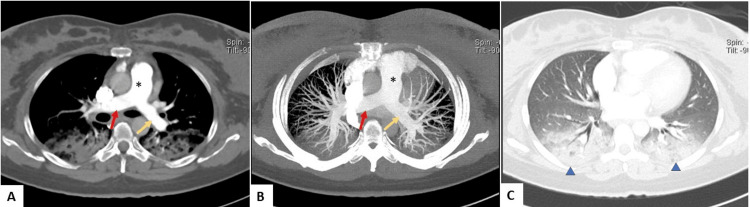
CT imaging: pulmonary angiography. (A) Axial pulmonary angiogram. (B) Axial maximum intensity projection. (C) Axial lung window Normal opacification of the main pulmonary trunk (asterisk), right pulmonary artery (red arrow), and left pulmonary artery (yellow arrow), showing no pulmonary embolism. Bilateral basal pulmonary densities mostly represent postanesthesia atelectasis (blue arrowheads)
